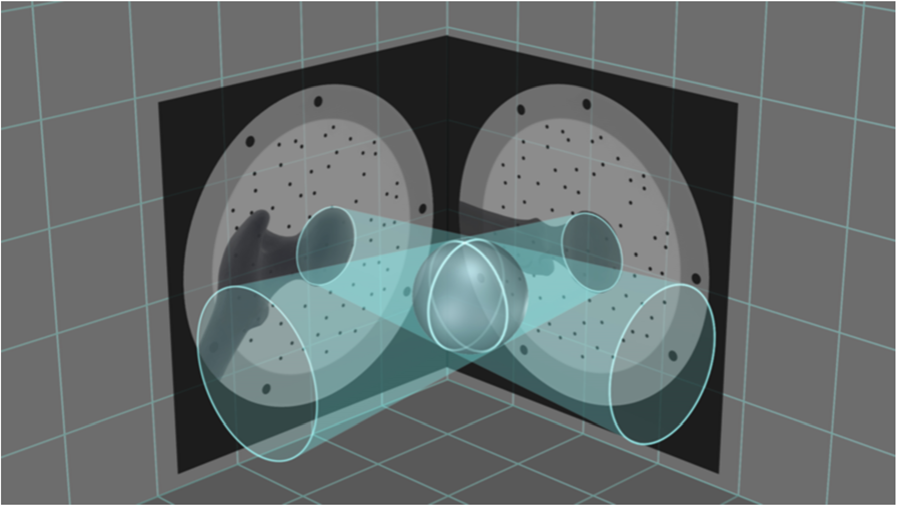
Principle: generation of a 3D model of the anatomy from two 2D X-ray images.
